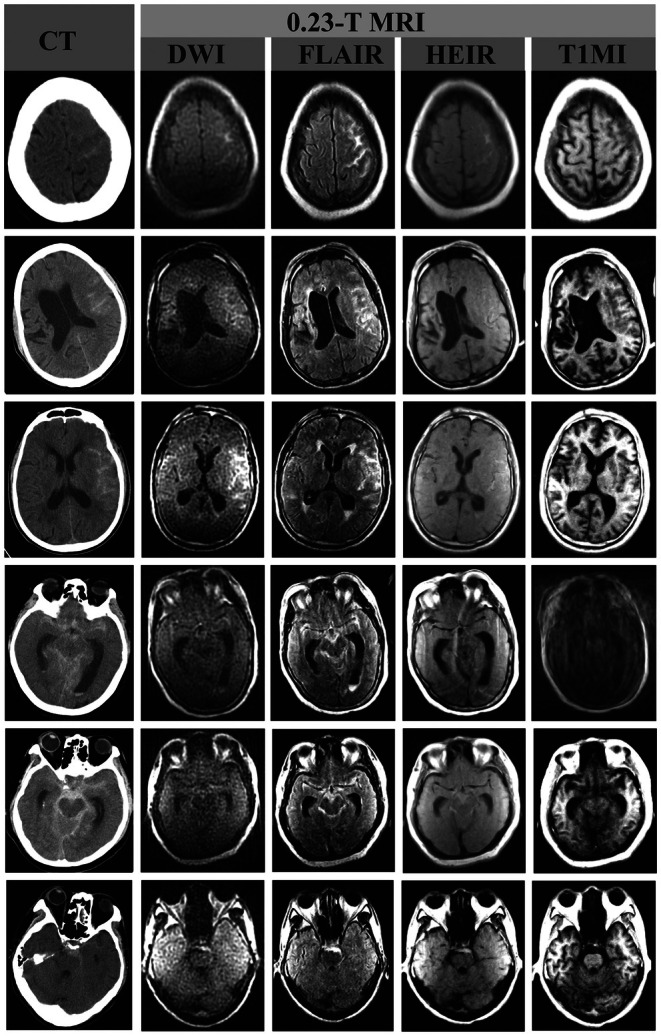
Representative cases of subarachnoid hemorrhage. CT, computed tomography; DWI, diffusion‐weighted imaging; FLAIR, fluid‐attenuated inversion recovery; HEIR, hematoma enhanced inversion recovery; mLF‐MRI, mobile low‐field magnetic resonance imaging; T1WI, T1‐weighted imaging.
